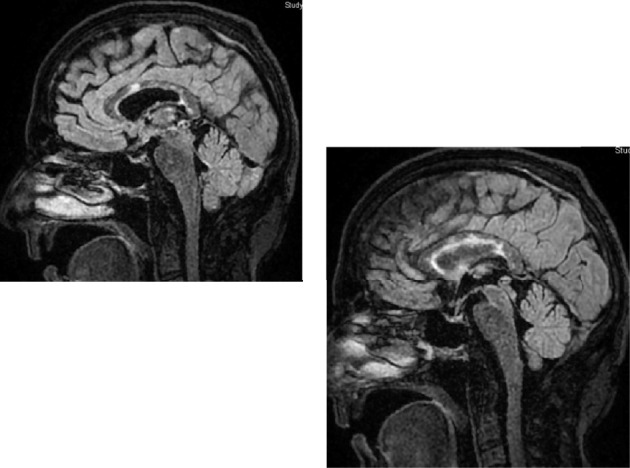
MRI findings with strokes along the body and splenium of the corpus collosum in the fluid attenuated inversion recovery (FLAIR) and T2 weighted MRI sequences, best viewed on sagittal sequences, with high signal regions that are well demarcated and spherical in shape, or referred to as “snowball strokes.”
